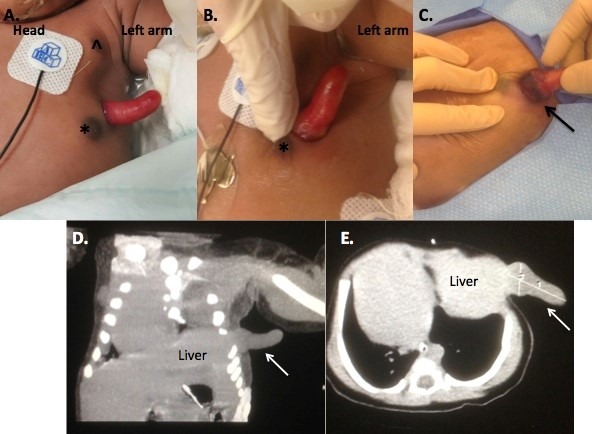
Figure 1: A. Appearance of the exophytic mass on initial examination. The mass was located on the left lateral chest between the normal (^) and accessory (*) left nipples. B. Upon close inspection, the mass was pink with a moist surface. C. Hyperemia and ischemia/necrosis at the base of the mass (black arrow) on day of life two. Coronal (D) and axial image (E) from computed tomography scan demonstrating extension of this mass from the liver (white arrow).
